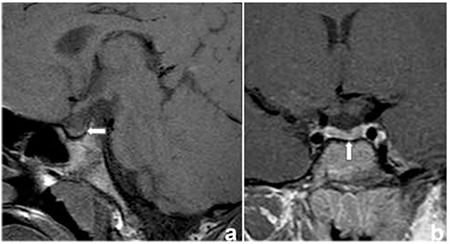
Sagittal pre-contrast (a) and coronal post-contrast (b) T1-weighted magnetic resonance images showing a small adenohypophysis and decreased normal hyperintense signal of the neurohypophysis
